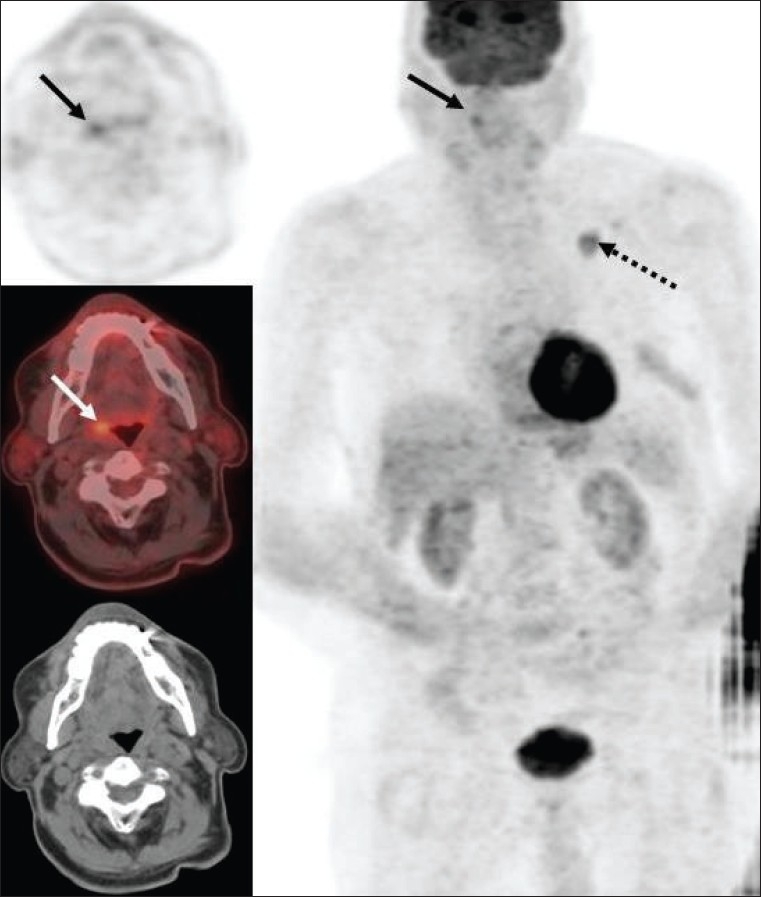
Axial PET (top left), fused PET/CT (middle left) and CT scan (bottom left) images at the level of the tonsils, with an MIP image (on right), from a PET study of an 80-year-old man with a left lung cancer that shows increased FDG uptake (dotted arrow). Incidentally noted is increased uptake in the right tonsil (arrows), which was found to be due to chronic inflammation
